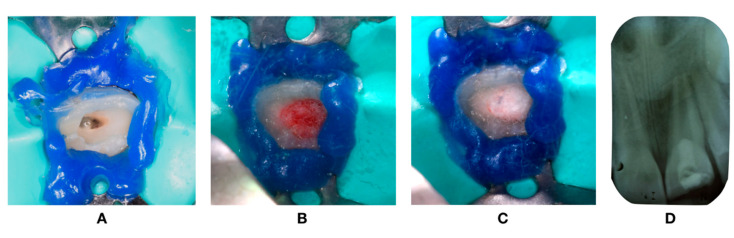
Intra-oral photography. (A) Coronary opening. (B) Blood clot. (C) MTA placed apical to cementoenamel junction. (D) Periapical radiograph after REP procedure.
